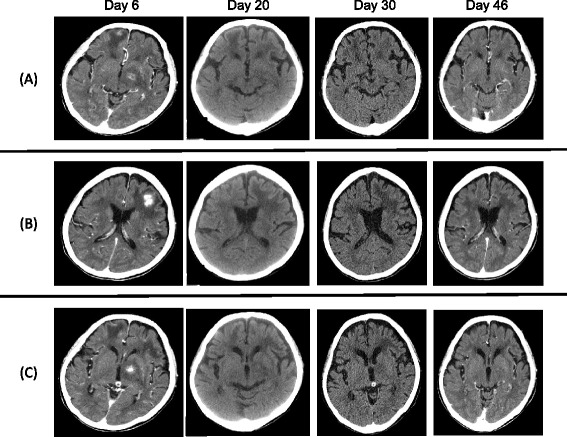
Cranial computed tomography scan after the withdrawal of methotrexate. The masses of (A) right frontal lobe, (B) left frontal lobe, and (C) left nucleus basalis were gradually reduced.
